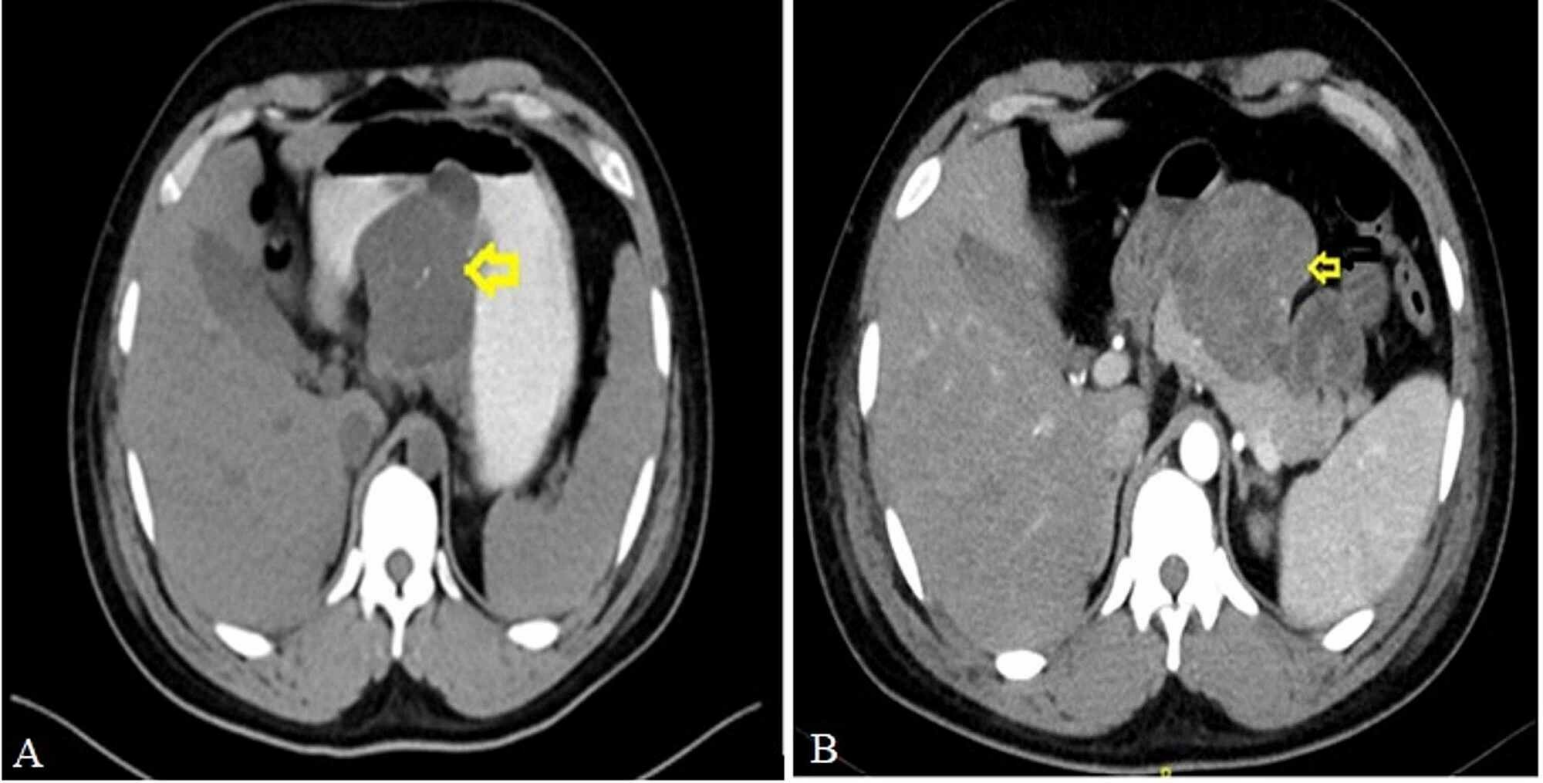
Computed tomography abdomen showing tumor in the lesser sac (arrow) involving posterior wall of stomach and pancreas. (A) With oral contrast. (B) Without oral contrast.
